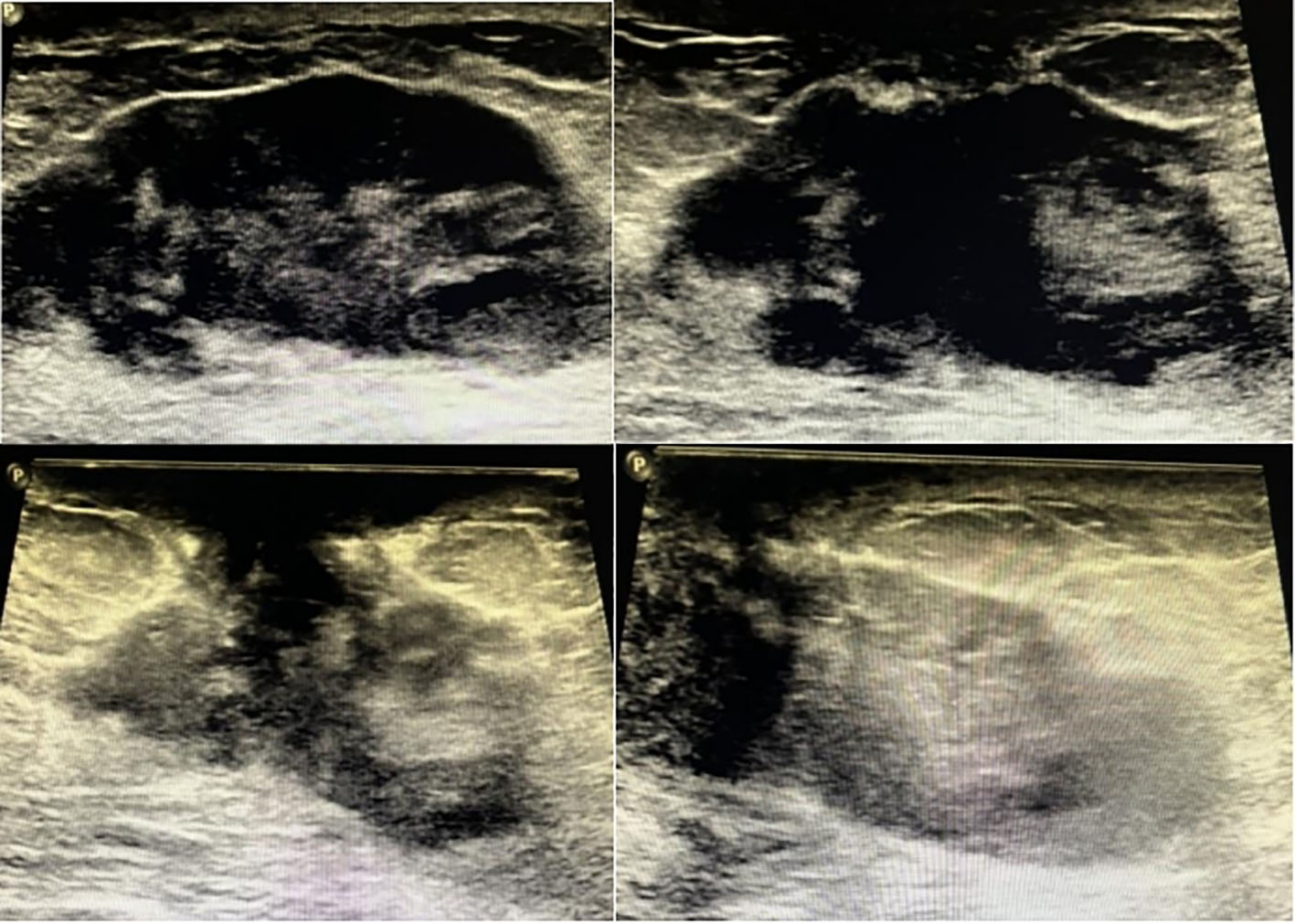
Sonography of the patient of breast desmoid-type fibromatosis in different sections of scan.
